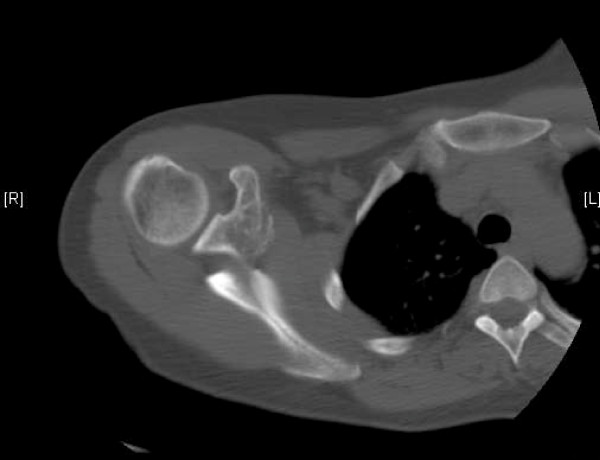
CT scan showing the metastatic lesion at the base of the coracoid.
